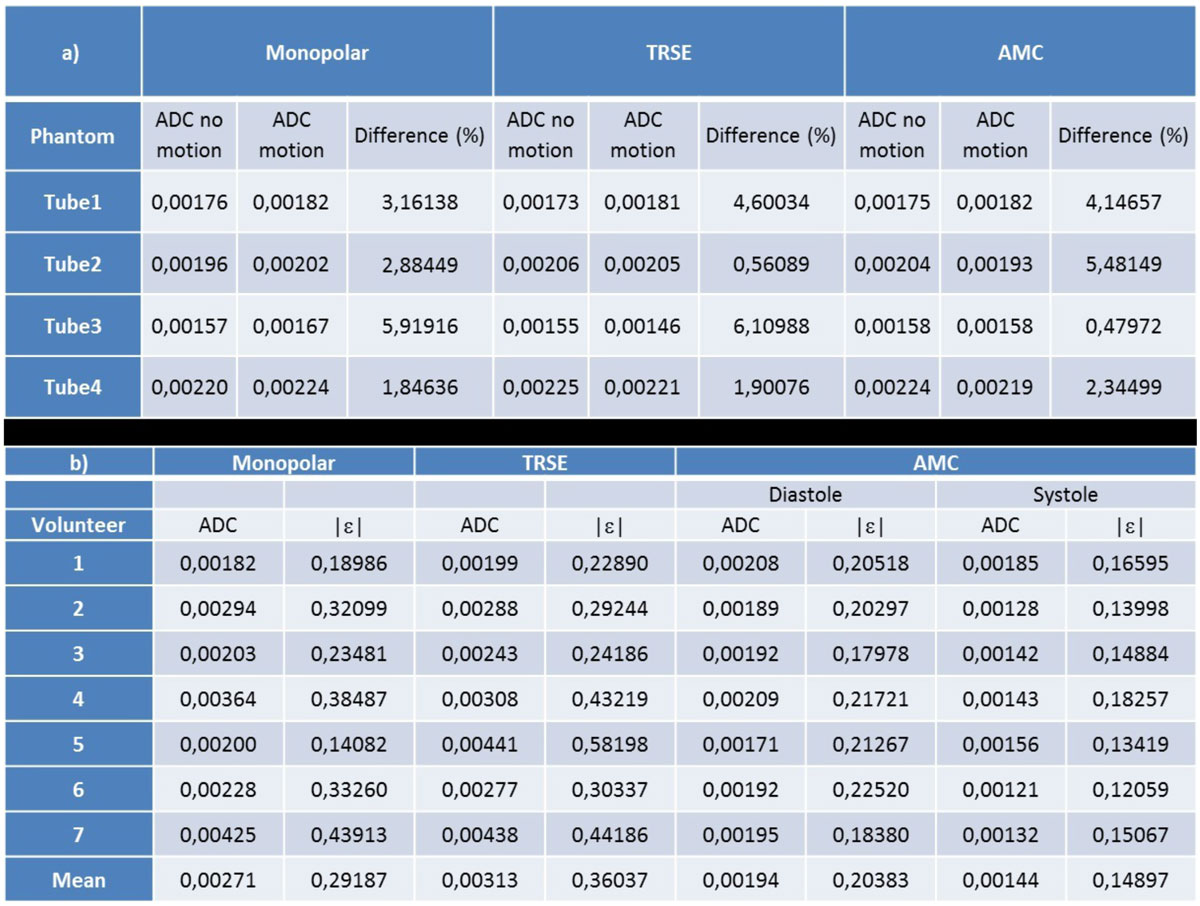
a) ADC calculation (mm^2^/s) extracted from mono-exponential fitting on the 8 values for the 4 tubes with and without motion. a) ADC measurement (mm^2^/s) extract from trace data and mean artifact quantification ε (no unit, mean over the 6 DWI directions) for the 7 volunteers.
